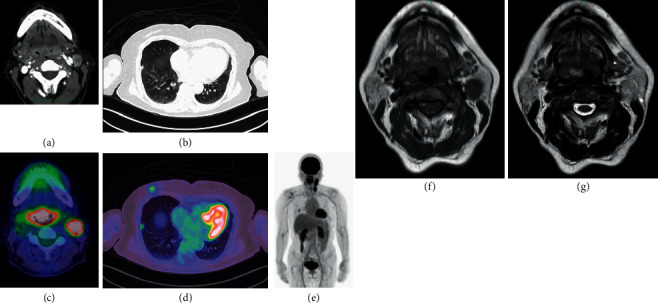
(a) Neck CT showed a tumor at the base of the tongue and left cervical lymph node metastasis. (b) Chest CT showed a nodule in the right inferior lobe of the lung and a postoperative scar lesion derived from breast cancer on the right chest wall. (c) PET-CT showed marked glucose uptake by the base of the tongue (maximum standardized uptake value (SUV max) early: 16.35, delayed: 17.90), the left cervical lymph nodes (SUV max early: 15∼17, delayed: 17∼19), (d) the scar on the right chest wall (SUV max early: 3.47, delayed: 3.19), and the nodule in the right lung (SUV max early: 2.09, delayed: 2.42). (e) There was no other apparent distant metastasis. (f, g) Neck MRI T1-weighted imaging (WI) and T2WI showed that the tumor at the base of the tongue and the left cervical lymph nodes exhibited slightly higher intensity than the surrounding muscles.
